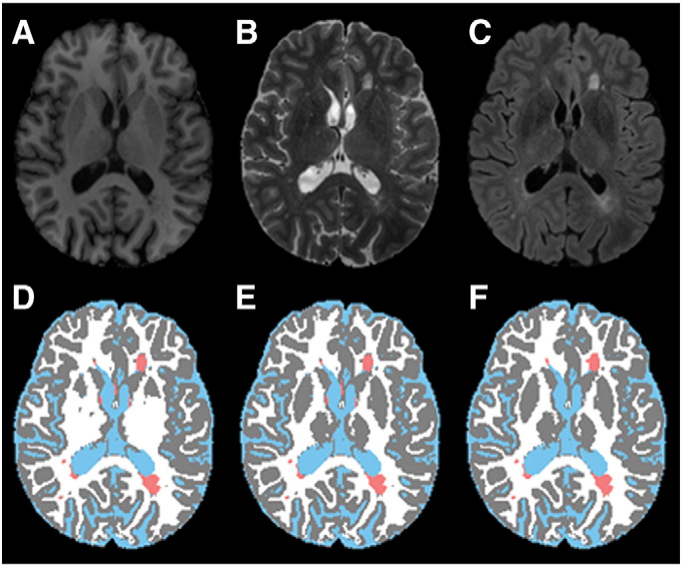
Re-classification of dGM structures. A: T1; B T2; C: FLAIR; D: segmented images; E: following the re-classification of dGM structures using deformed template and its anatomical image; and F: image with corrected lesions. Color scheme is same as Fig. 2.
